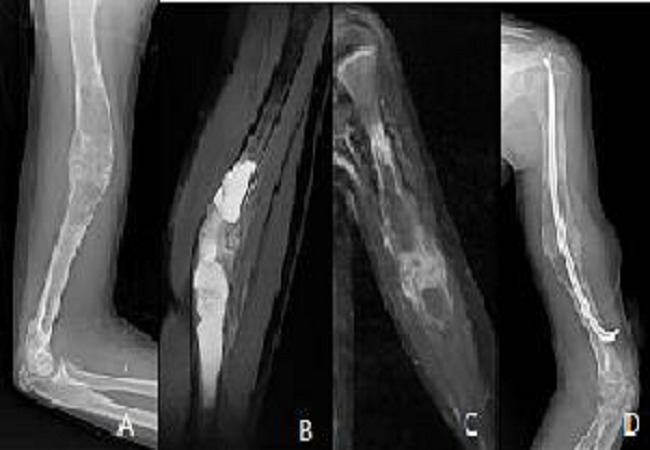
Radiograph shows osteolytic lesion very limited of the upper end of tibia
